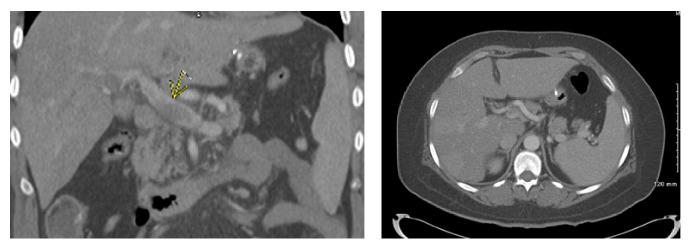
Contrast enhanced axial and coronal computed tomographic images of the abdomen. There is a lack of enhancement in the main portal vein (arrow) due to thrombosis.
